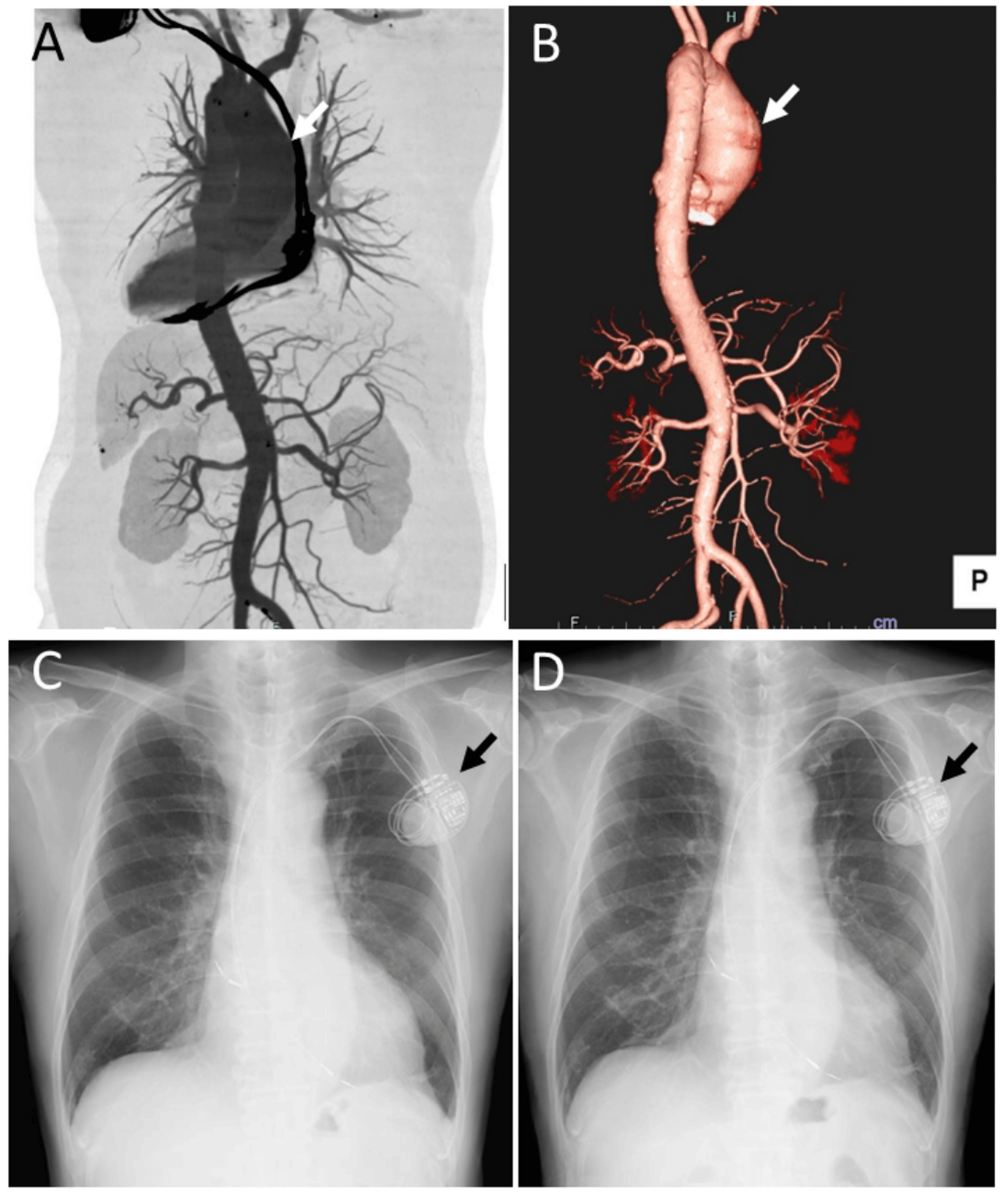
CT angiography at age 65 years and plain chest radiographs at age 71 years CT angiography (posterior views) at age 65 years (A, B) showing a fusiform ascending aortic aneurysm with a diameter of 51 mm (arrows). Plain chest radiographs at age 71 years, obtained one month before presentation (C) and at the onset (D) of right CRVO, showing no change in cardiomegaly on the two occasions. Arrows indicate the cardiac pacemaker.
